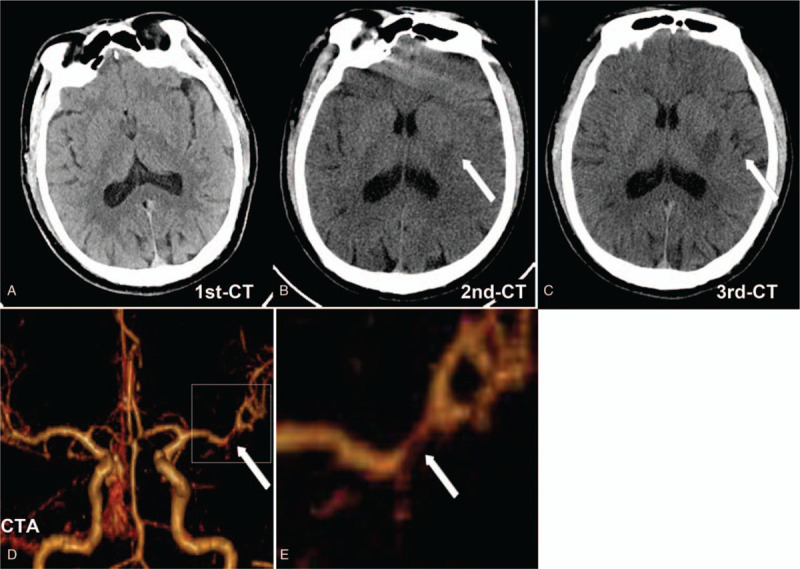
Brain CT and CTA of the patient. (A) Brain CT at 5 hours after the first episode of TIAs (the third TIAs) shows normal. (B) Brain CT at 14 hours after the first episode of TIAs (repeat CT before r-tPA thrombolysis) shows small acute infarcts in the right putamen (white arrow). (C) Brain CT at 39 hours after the first episode of TIAs (24 hours post r-tPA thrombolysis) shows larger acute infarcts in the right putamen (white arrow) and no bleeding. (D) Brain CTA shows M1 segment stenosis of the left middle cerebral artery (white arrow). (E) The higher magnification of M1 segment stenosis(white arrow, Fig.2 E is the boxed area in Fig. 2D). CT = computed tomography, CTA = computed tomography angiography, r-tPA = recombinant tissue plasminogen, TIAs = transient ischemic attacks.
